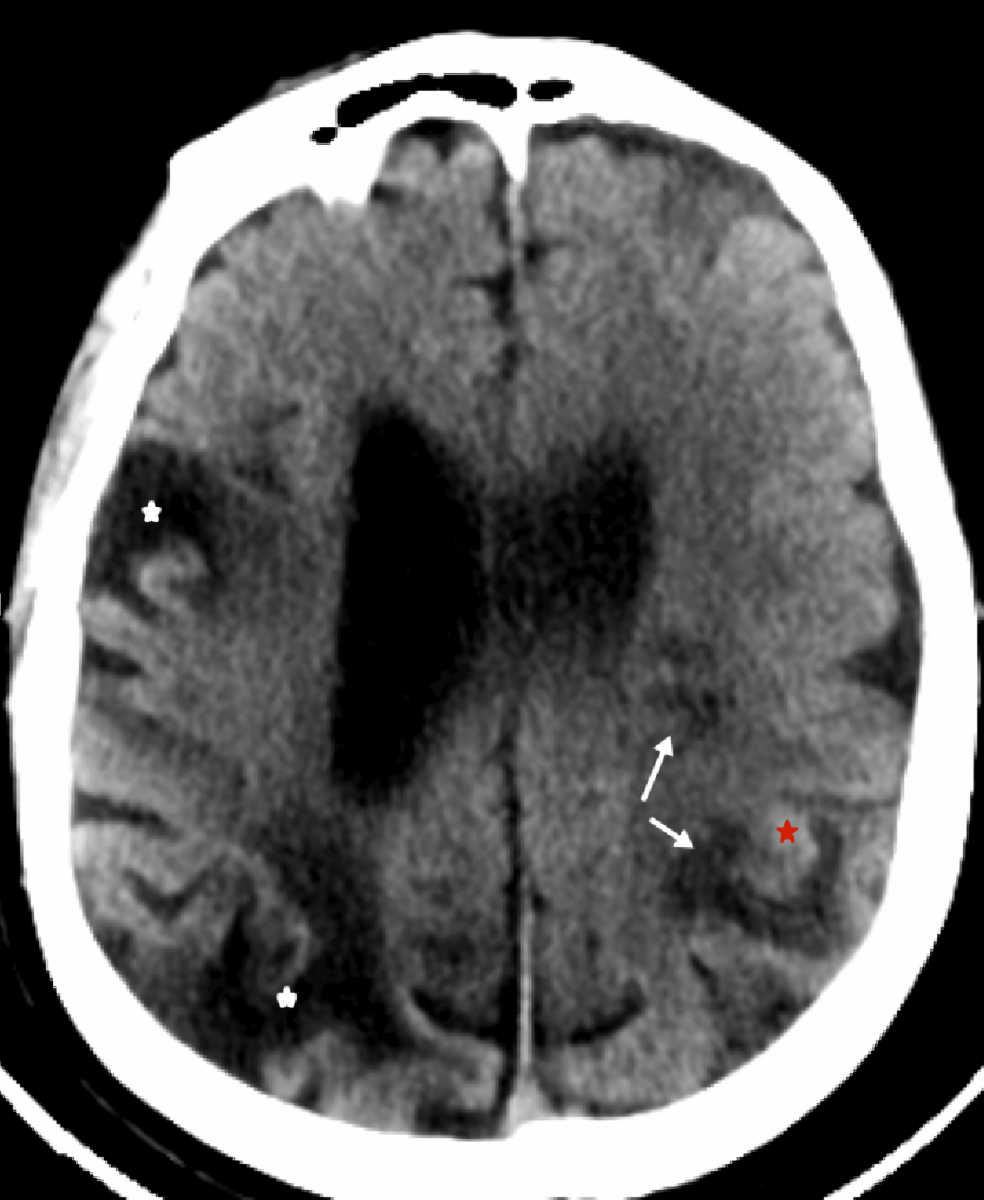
Non-contrast computed tomography (CT) scan showed subacute ischemic lesions in the left middle cerebral artery (MCA) territory (white arrows), with hemorrhagic transformation (red star) and sequelae of right MCA infarction (white stars).
